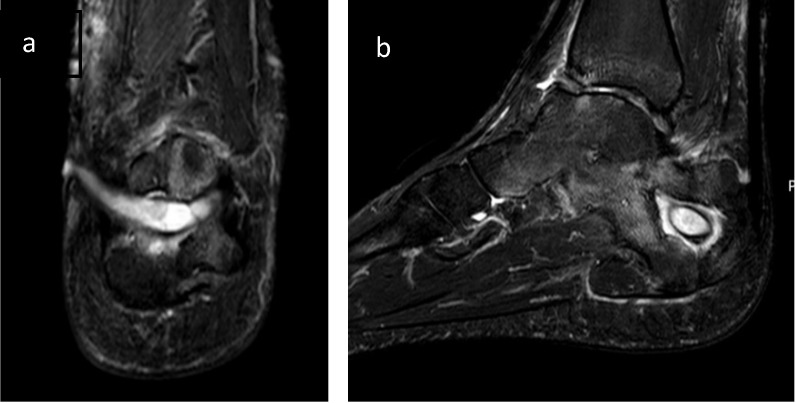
1a: Coronal MRI showing central abscess with sinus extending to the medial side. There is no subperiosteal extension of the abscess. This infection followed internal fixation of a closed, complex calcaneal fracture. 1b: Sagittal MRI showing the classical 'corona' sign of osteomyelitis.
